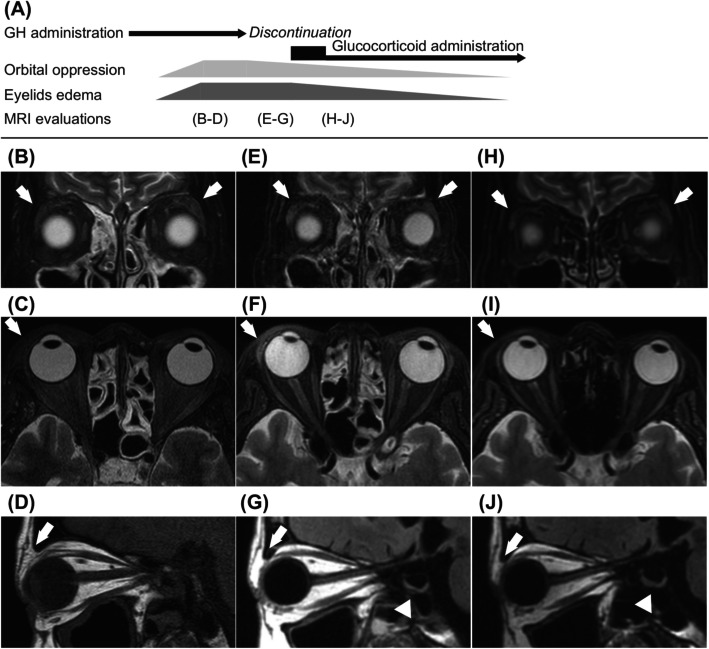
Sequential change of thyroid-related ophthalmopathy demonstrated by magnetic resonance imaging. The clinical course of growth hormone and glucocorticoid therapy, symptoms of Graves’ ophthalmopathy, and points of MRI evaluations are displayed (A). The bilateral lachrymal glands swelling, especially in left side (B), and right eyelid swelling (C, D) indicating thyroid-related ophthalmopathy after growth hormone replacement are shown. Minimal attenuation of swellings in lachrymal glands is shown after growth hormone discontinuation (E), while edematous change in right eyelid persisted (F, G). Glucocorticoid therapies improve further bilateral lachrymal glans and right eyelid edema (H, I, J). (B, E, H): frontal section of T2 weighted image. (C, F, I): horizontal section of T2 weighted image (C: short T1 inversion recovery; F and I: iterative decomposition of water and fat with echo asymmetry and least squares estimation). (D, G, J): sagittal section image (D: T1 weighted image; G and J: fluid-attenuated inversion recovery). Arrows indicate bilateral lachrymal gland (B, E, H) and right eyelids (C, D, F, G, I, J). Arrowheads (G, J) indicate pituitary gland exhibiting a mild compression form
